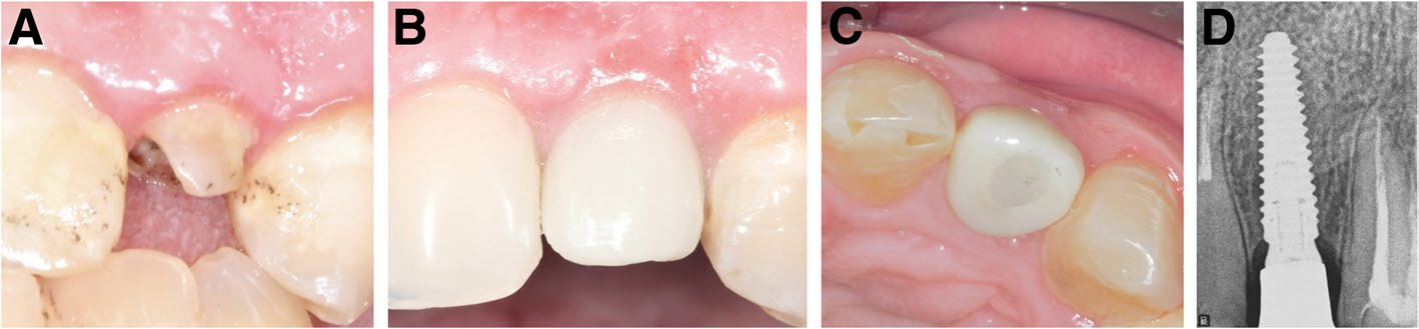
A-D: Photos and a radiograph of case #6 replacing the maxillary left lateral incisor. a Preoperative frontal view; b frontal view of the definitive crown at 12 months postloading; c incisal view of the definitive crown at 12 months postloading; and (d) periapical radiograph at 12 months postloading
